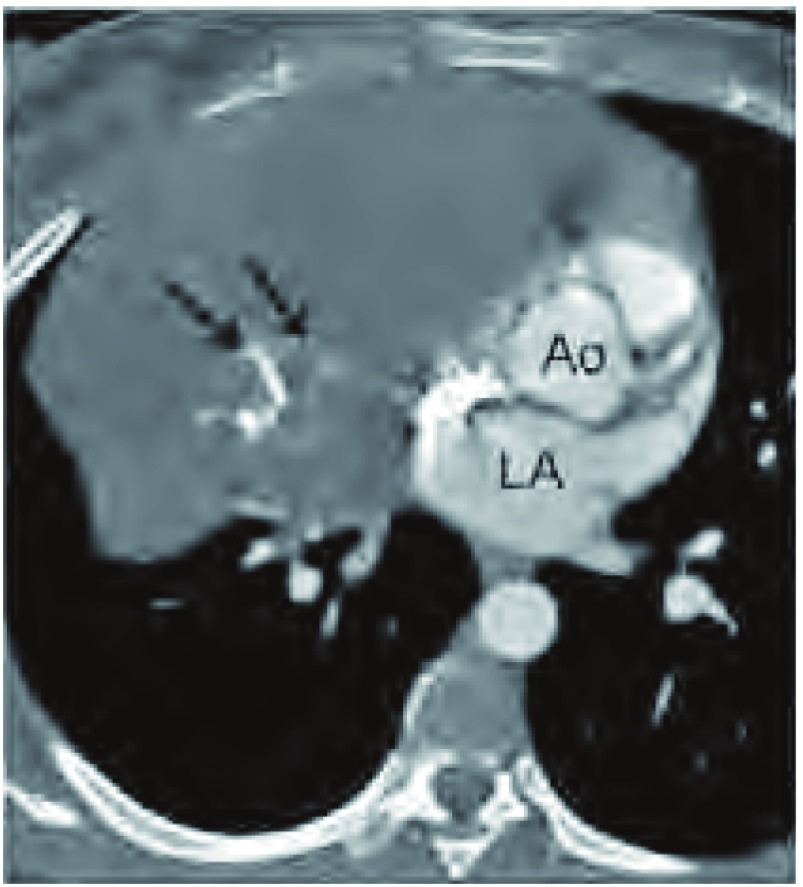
钙化。胸部增强CT（软组织窗）显示，曲线样钙化（黑箭头所示），其形态与高密度的血管形态不同，多数密度高于左心房（LA）和升主动脉（Ao）。 Calcification. Contrast-enhanced chest computed tomography (CT) (soft tissue window) at levels set between bone and mediastinal window. Curvilinear high-density regions (black arrows) are due to calcifications as they do not conform to the expected morphology of an opacified blood vessel, and most are of higher attenuation than opacified blood in the left atrium (LA) and ascending aorta (Ao).
